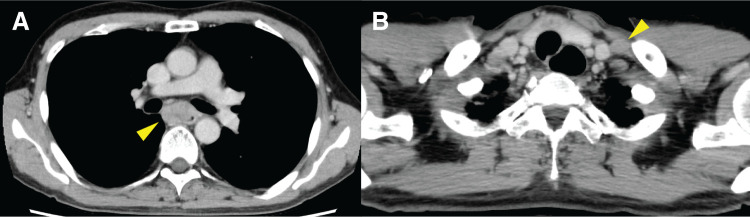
Contrast-enhanced CT before FP plus pembrolizumab. (A) Irregular wall thickening with contrast enhancement, suggesting infiltration into the left main bronchus (yellow arrow). (B) Enlarged lymph nodes in the paraesophageal and the left supraclavicular areas (yellow arrow).
